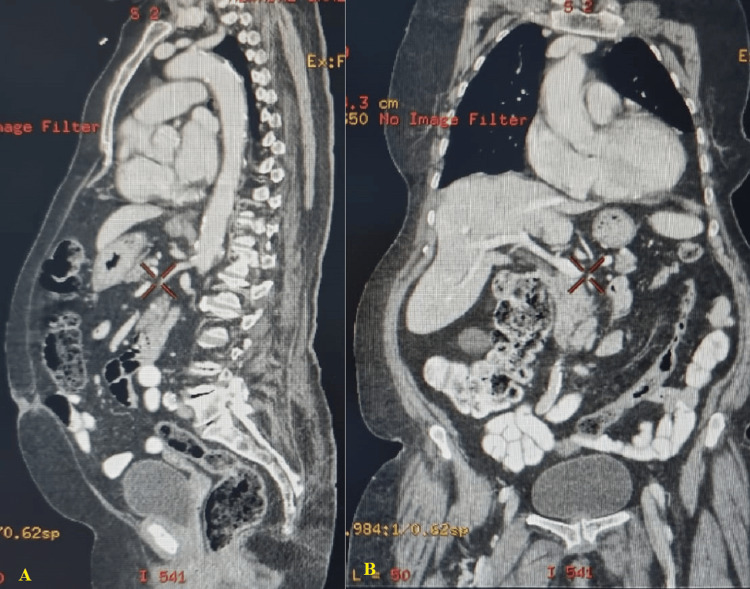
Follow-up abdominal CT scan with contrast three months after treatment revealed a normal urinary bladder without any abnormality or intraluminal lesion A: lateral view; B: anterior view CT: computed tomography
